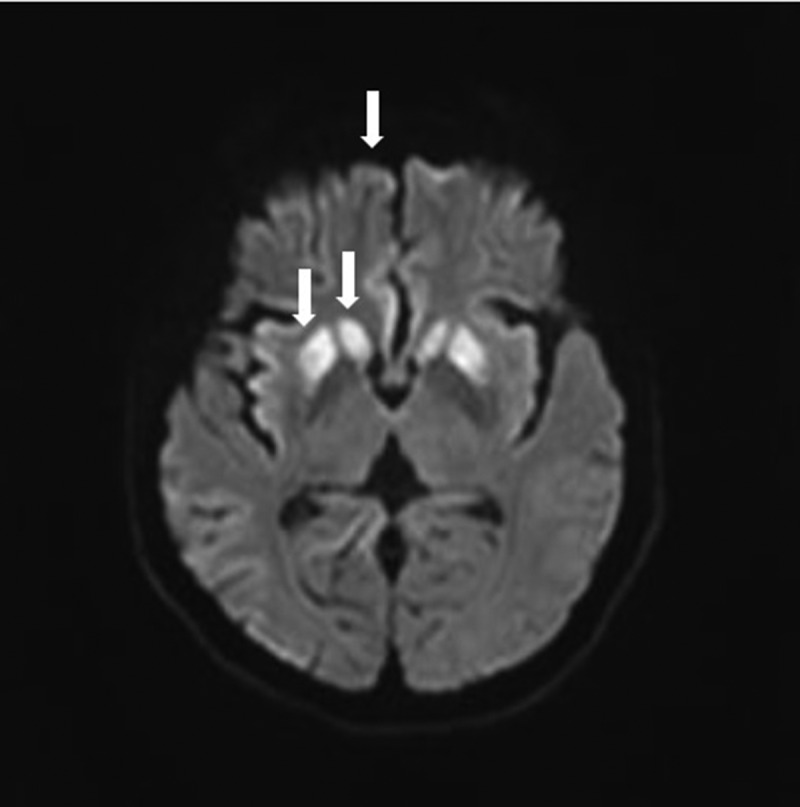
MRI with DWI image of the patient.
